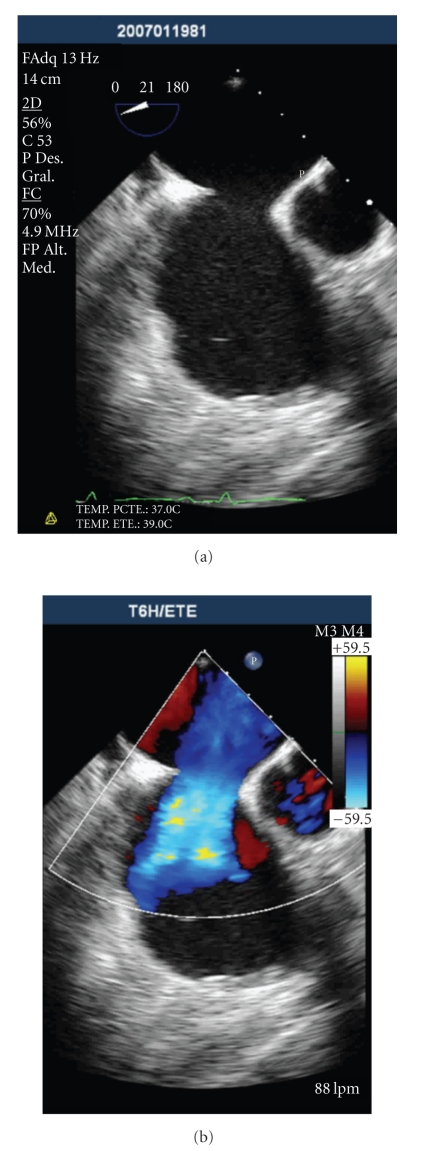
Transesophageal echocardiography showing interatrial communication with severe (a)–(b) shunt.
